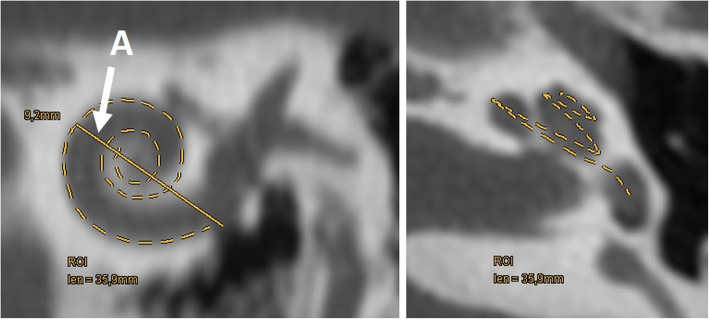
Evaluation of cochlear duct length (CDL) using the formula CDL = 4.16A–2.7 and a 3D segmentation (dashed line). HRCT paracoronal image on the left shows distance A (arrow) from the center of the round window to the far most extension of the basal turn, which measures 9.2 mm. According to the formula the cochlea duct has a length of 35.6 mm. The dashed line in the HRCT paracoronal image and the HRCT paraaxial image on the right shows the 3D segmented cochlear duct which measures 35.9 mm
